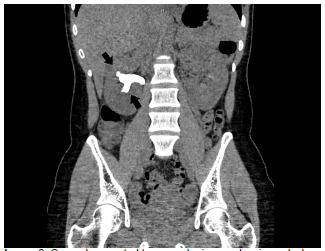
Coronal computed tomography image showing calculus filling entirety of right renal pelvis (arrows).
